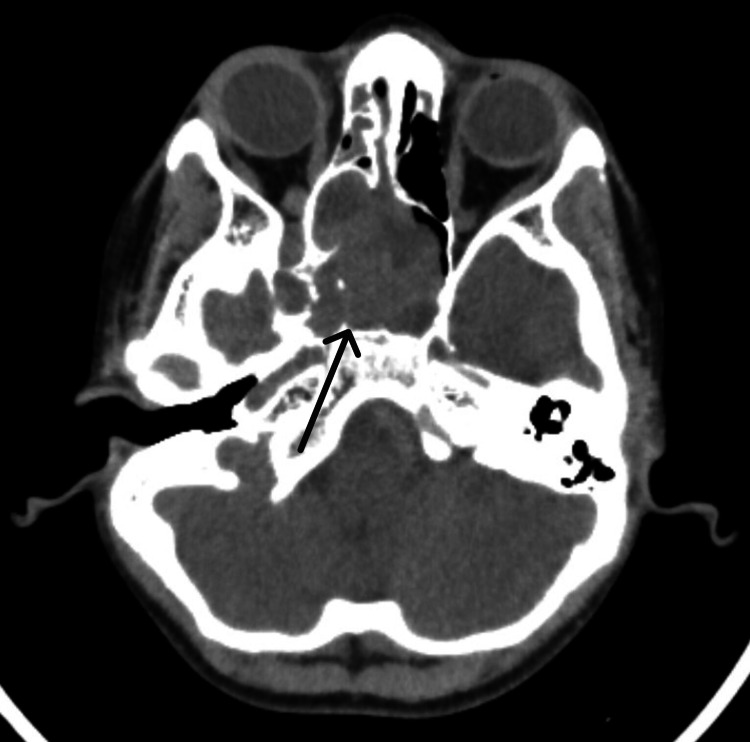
Preoperative CT scan of patient number 6 showing stage Ⅲb juvenile nasopharyngeal angiofibroma
